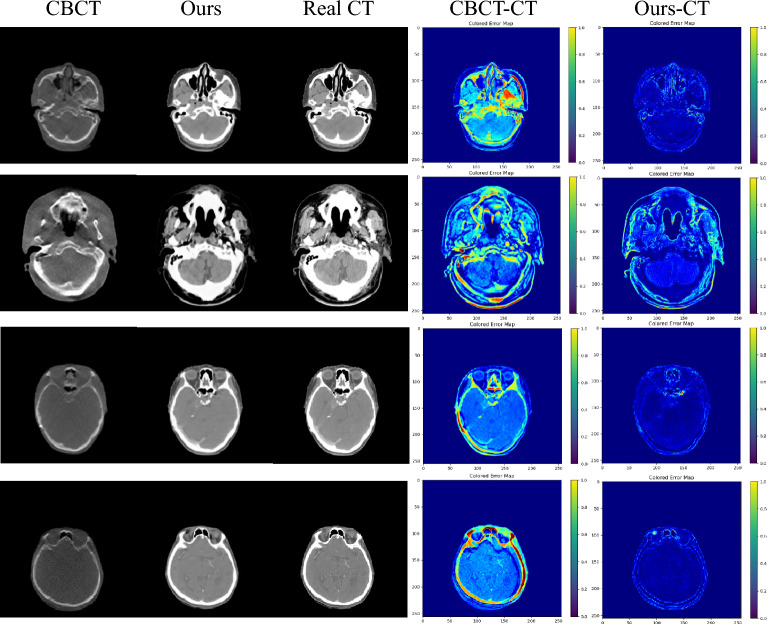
Error plots of CBCT vs. CT and the method of this paper vs. CT under the H &N dataset.
